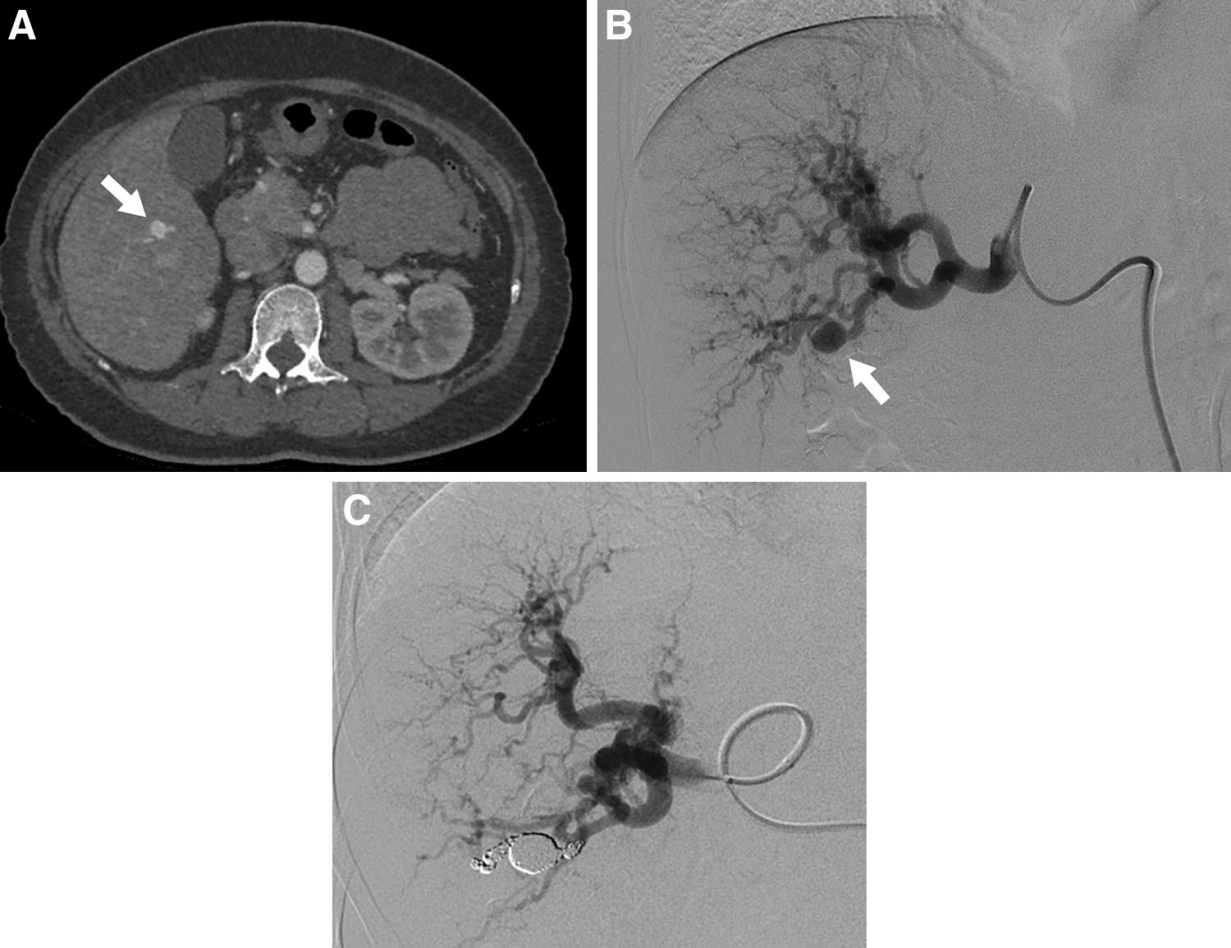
Hepatic artery aneurysm in 53-year-old woman with hereditary hemorrhagic telangiectasia. a The arterial phase of a contrast-enhanced CT scan reveals a hepatic artery aneurysm (arrow) located in the posterior segment of the liver. b Digital subtraction angiography of the hepatic artery demonstrates a saccular aneurysm (arrow) in the posterior branch of hepatic artery. c Postprocedural hepatic digital subtraction angiography shows complete obstruction of the aneurysm as well as the inflow and outflow arteries
